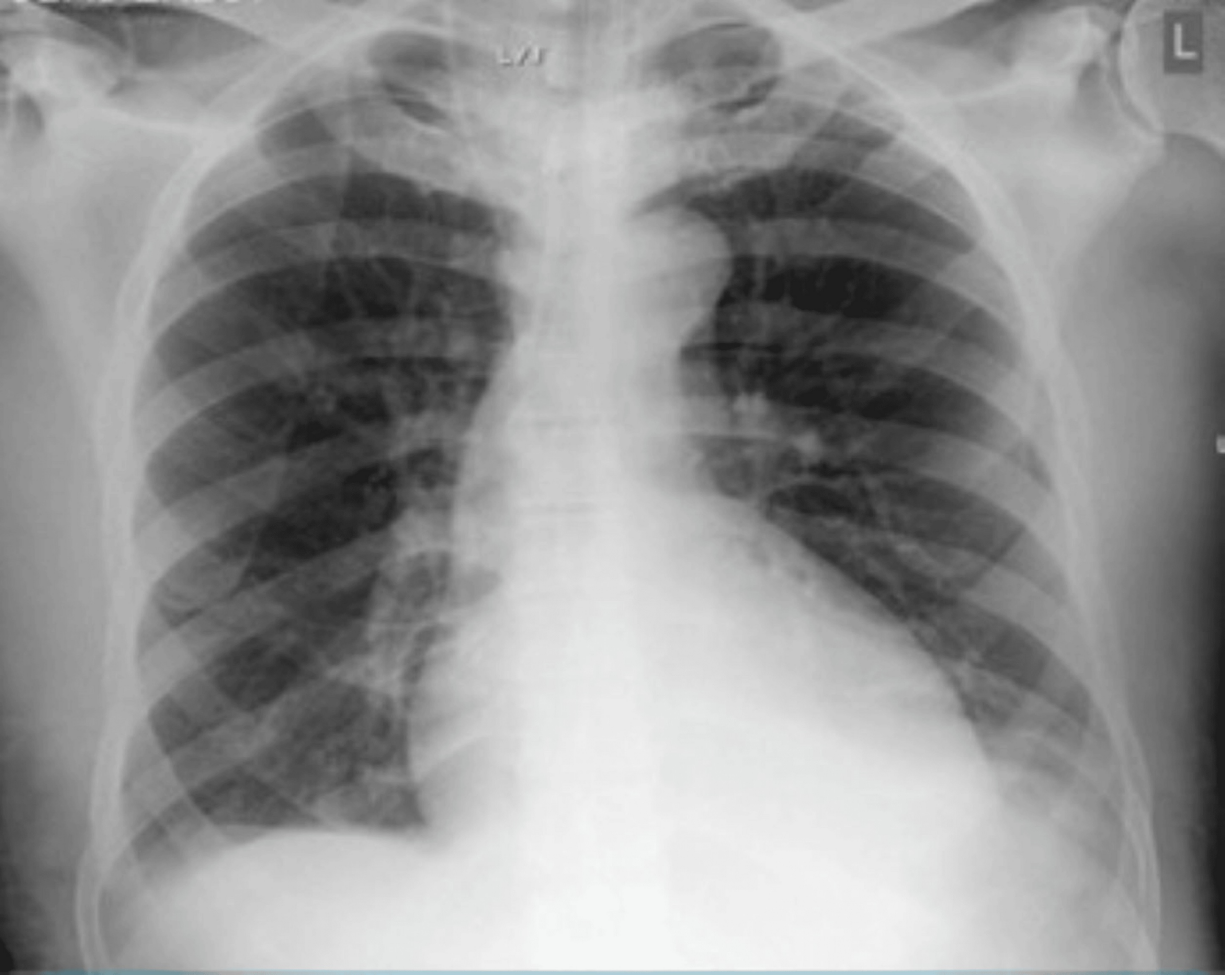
Chest X-ray of Case 1. This is the chest X-ray of Case 1 showing opacifications in the right mid to lower zones and opacification in the left mid-zone.
